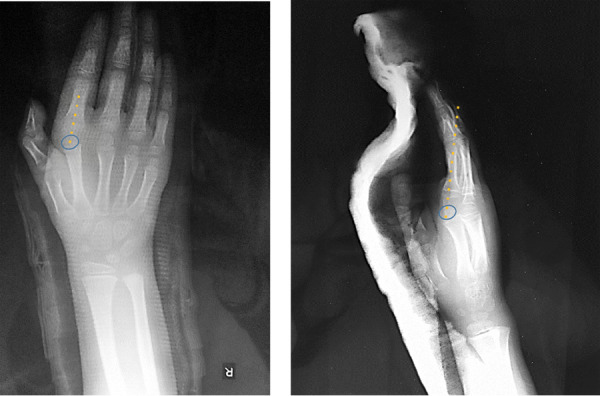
Postoperative X-Ray images.
